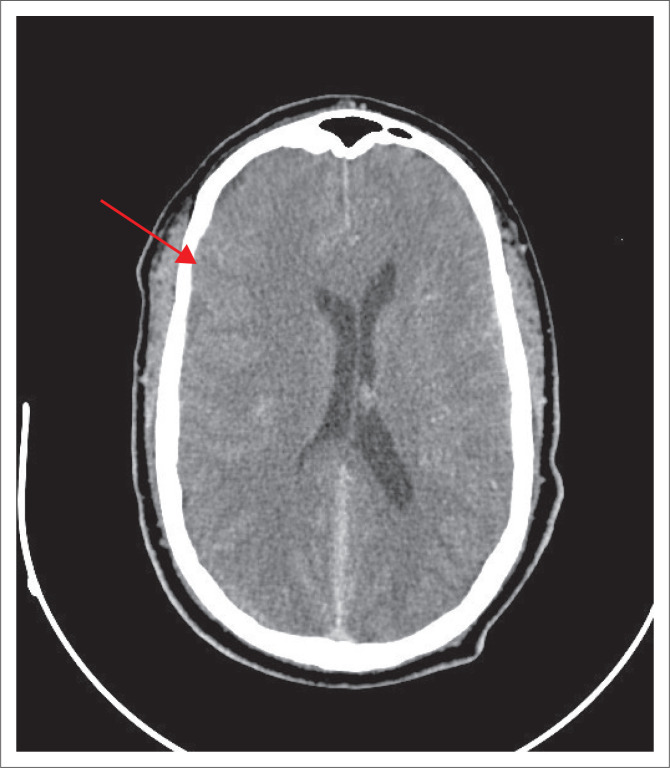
Computed tomography of the brain showing midline shift of 4 mm with evidence of a right subdural haemorrhage.
